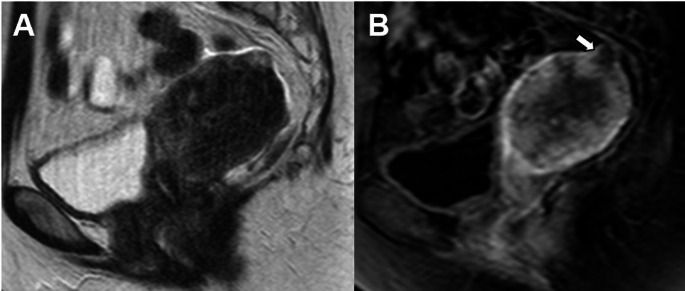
Stage IIIA2 serous carcinoma in a 70-year-old woman. A Sagittal T2-weighted fast spin-echo (FSE) image (TR/TE: 4500/100 ms; slice thickness: 3 mm) and B sagittal contrast-enhanced 3D T1-weighted fat-suppressed LAVA sequence (TR/TE: 4.2/2.1 ms; slice thickness: 2 mm; post-gadolinium, delayed phase at 4 min) show absent normal myometrial signal intensity and an irregular uterine contour at the fundus (arrows). Pathology confirmed serous carcinoma with involvement of the uterine serosa
